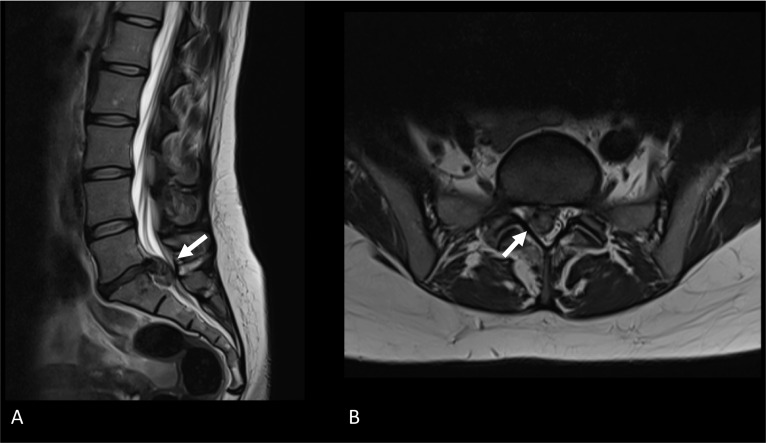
MRI of the lumbar spine demonstrates a large disk herniation on level L5–S1. A Sagittal T2-weighted MRI depicts disk protrusion at level L5–S1. A close relation with the cauda equina roots can be observed. B Axial T2-weighted MRI shows the protruding disk at level L5–S1 occupying part of the central zone and right subarticular zone. Cauda equina roots are displaced to the contralateral side of the dural sac
